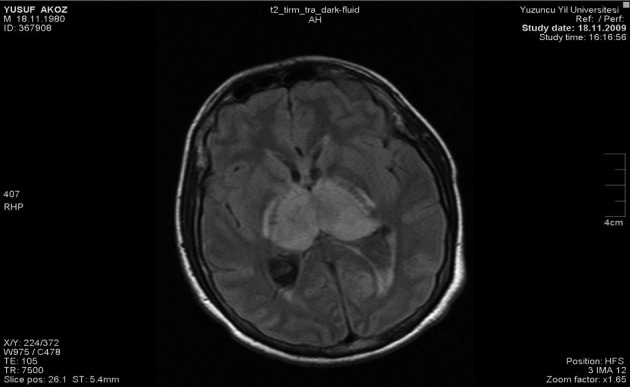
MRI of the cranium revealed infarct areas of posterior limb of capsula interna, right globus pallidus, left occipital lobe and bilateral thalamus.
